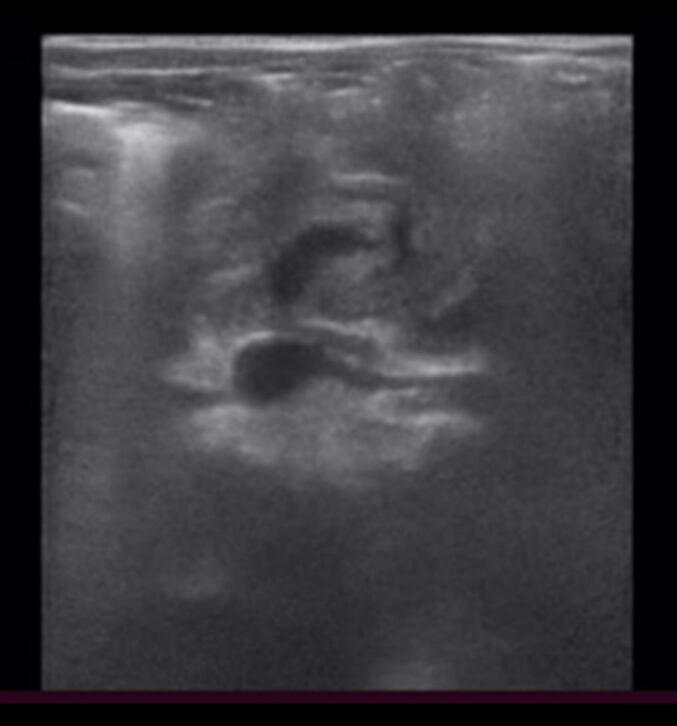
Grey scale ultrasound at the level of the upper abdomen showing whirling vessels and surrounding small bowel loops on transverse scan.
